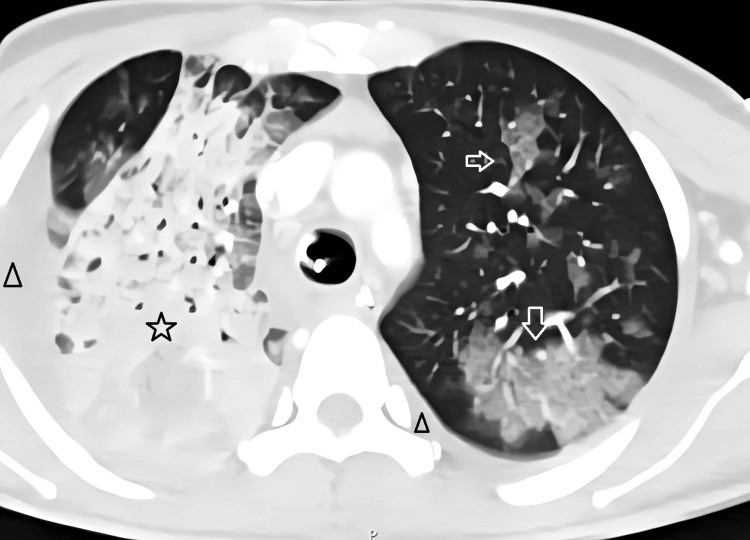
Second CT scan showing pleural effusion suggestive of bilateral empyema (triangles), extensive consolidation of the right upper lobe (star), and pronounced ground-glass opacities in various pulmonary lobes bilaterally (arrows). CT, Computed tomography
